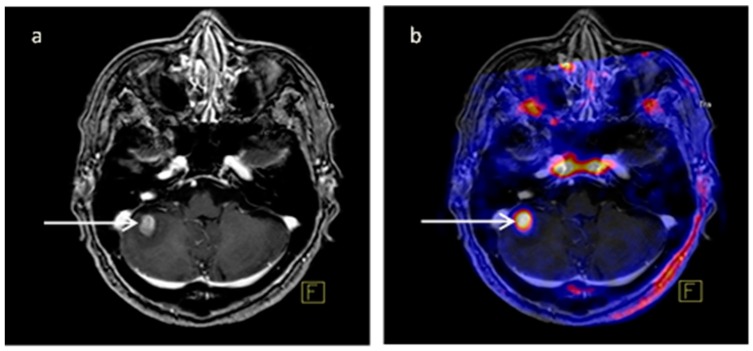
MRI-T1 post gadolinium sequence (a) and fused PET/MRI (b) images. Cerebellar metastatic lesion (arrows) 4 months after radiotherapy. Primary cancer site is breast. SUVmax, T/B, SUVpeak 2.62, 13.1 and 1.54 respectively. PV50% and PV75% 1.302 and 0,401 respectively.
